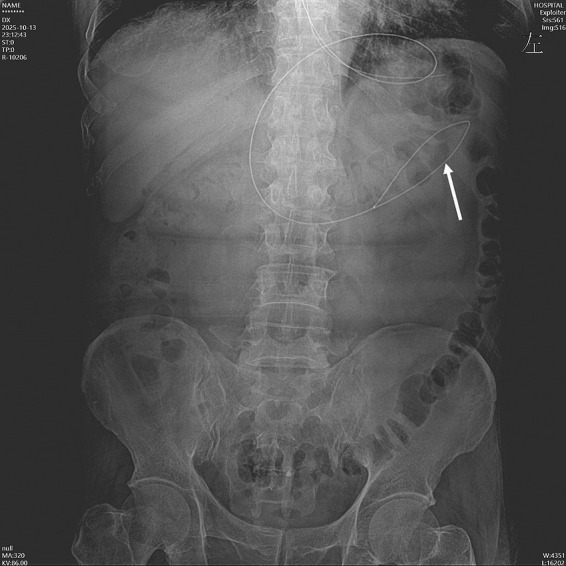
Radiographic confirmation of catheter placement. Abdominal X-ray showing the radiopaque hydrophilic-coated visualized nasojejunal tube passing through the stomach into the small intestine, with the distal tip located in the proximal jejunum (white arrow), confirming successful post-pyloric placement.
